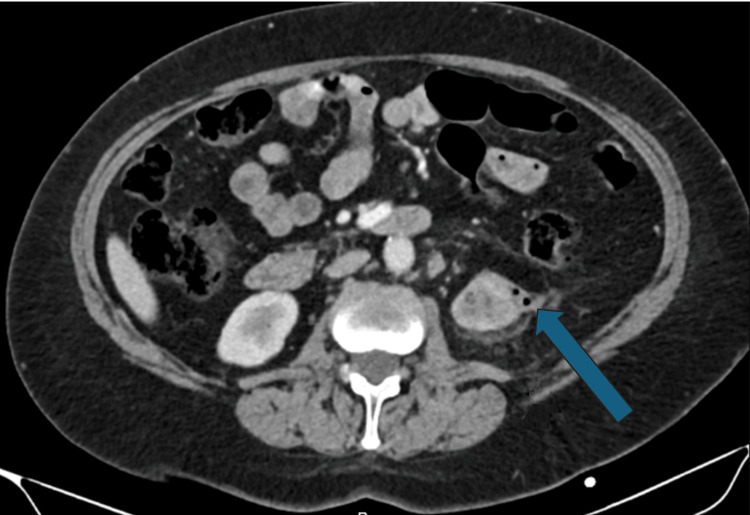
Axial non-contrast CT scan shows gas in the left kidney, indicated by the blue arrow, suggesting the presence of a fistulous connection to the bowel or emphysematous pyelonephritis.
